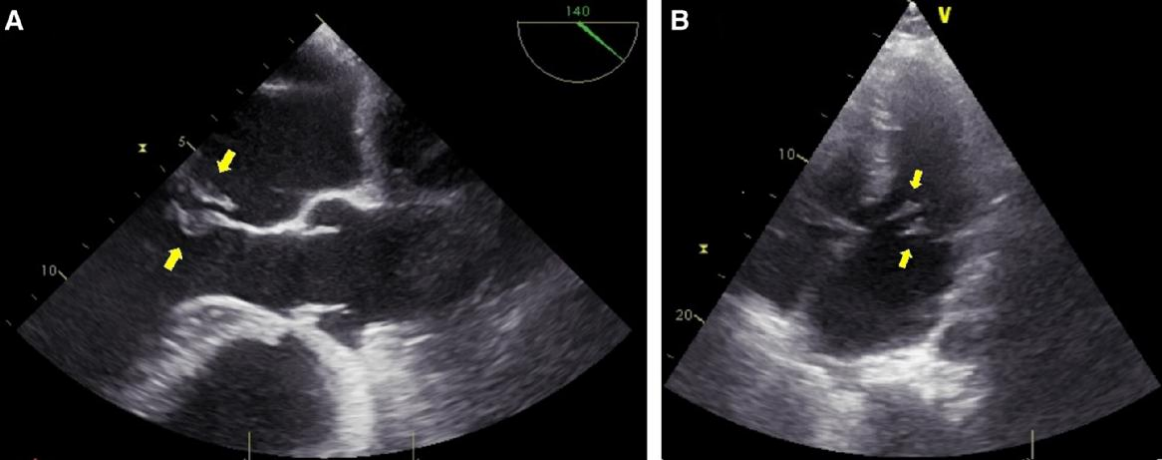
(*A* and *B*) Transoesophageal echocardiography mid-oesophageal aortic valve long-axis (ME AV LAX) and transthoracic echocardiogram apical 5C view at the time of admission showing vegetations on both leaflets of the mitral valve (arrows), with largest vegetation having the size of 8 × 3 mm.
